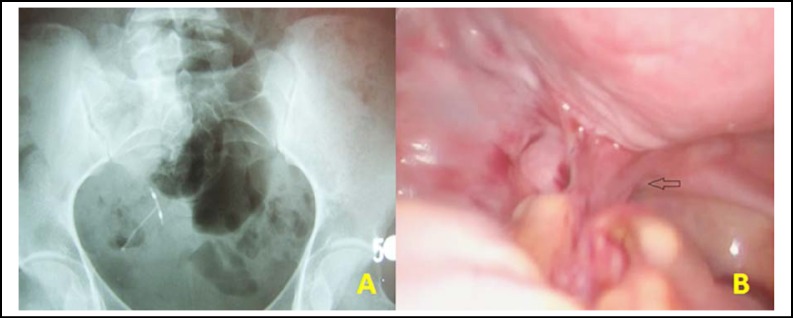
a- The X-ray image of dislocated IUD b-The laparoscopic view of dense adhesion between the left sacrouterine ligament and the sigmoid colon
